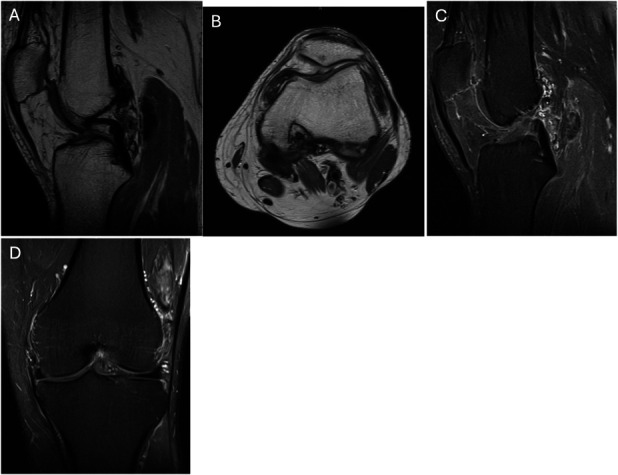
Imaging after neoadjuvant pexidartinib treatment. Fig. 4-A T1-weighted sagittal and axial (Fig. 4-B) with postcontrast, fat-saturated sagittal (Fig. 4-C) and coronal (Fig. 4-D) demonstrating substantial but incomplete disease response. There are persistent foci of TGCT anteriorly in the medial and lateral gutters, as well as posteriorly along the joint capsule and into the gastrocnemius muscle belly. The lytic defect of the posterior distal femur is clearly demonstrated. TGCT = tenosynovial giant cell tumor.
